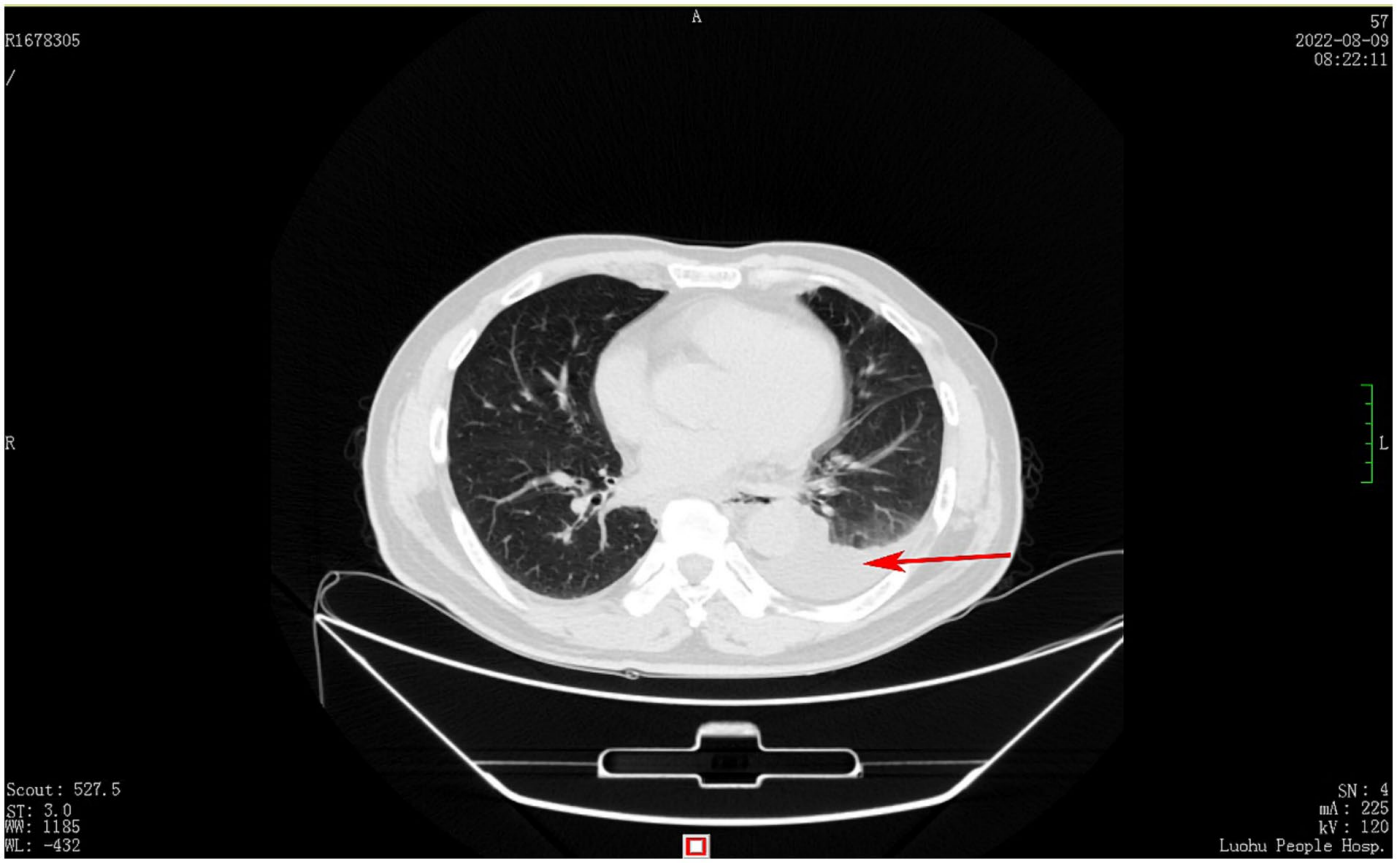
Chest computed tomography shows a left pleural effusion (red arrow).
